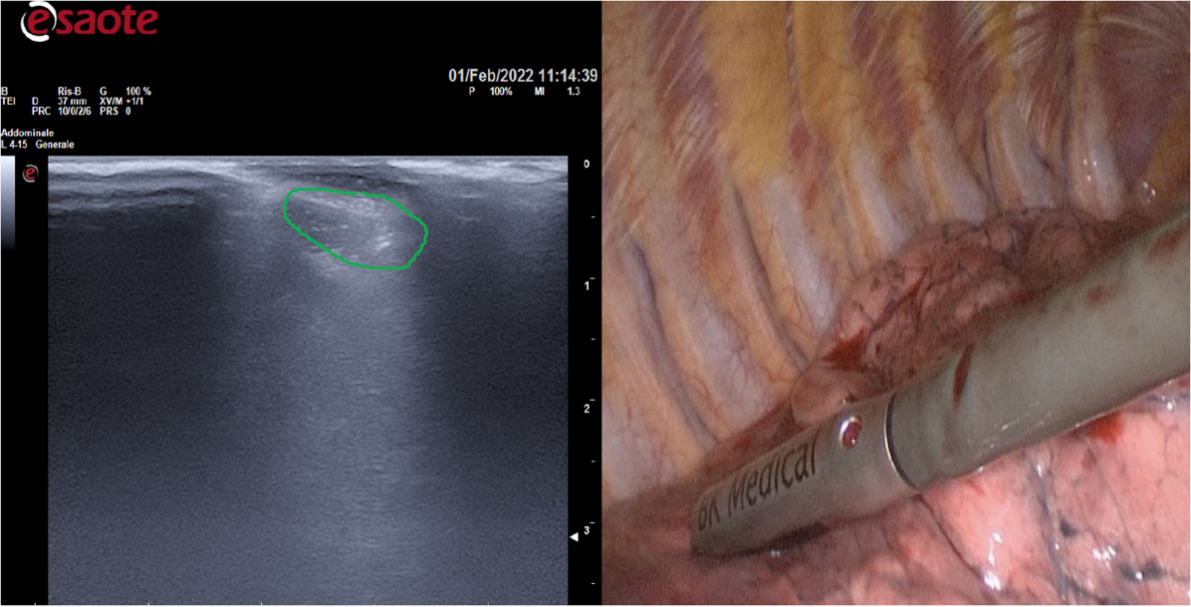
Intraoperative ultrasound can detect accurately the location and can define the characteristics of Ground-glass opacities.
